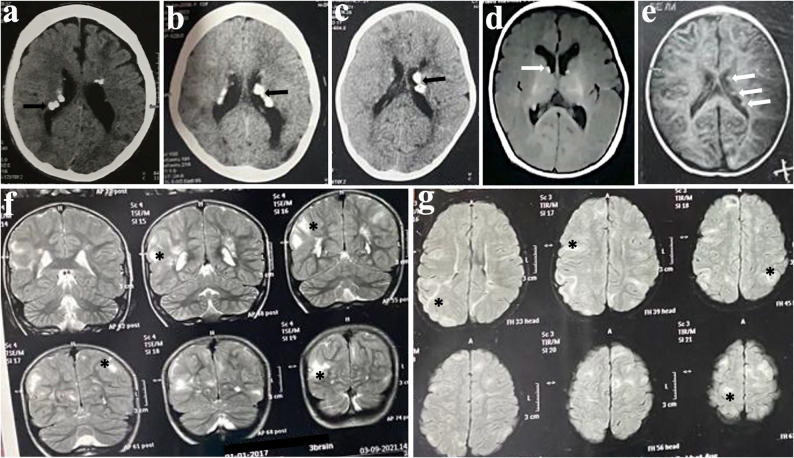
Representative brain imaging findings in patients with tuberous sclerosis complex. Brain axial CT (a – c), axial T1WI MRI (d, e), coronal T2WI (f), and axial GRE WI (g) sequences. Multiple subependymal nodules are observed along the lateral ventricular walls, particularly near the caudothalamic grooves, appearing as hyperdense lesions on axial CT images (a – c; black arrows) and isointense to hypointense on axial T1WI (d & e, white arrows). There are multiple cortical and subcortical tubers, appearing as hyperintense lesions (black asterisks) on coronal T2WI (f) and GRE WI (g) images with no blood signal
